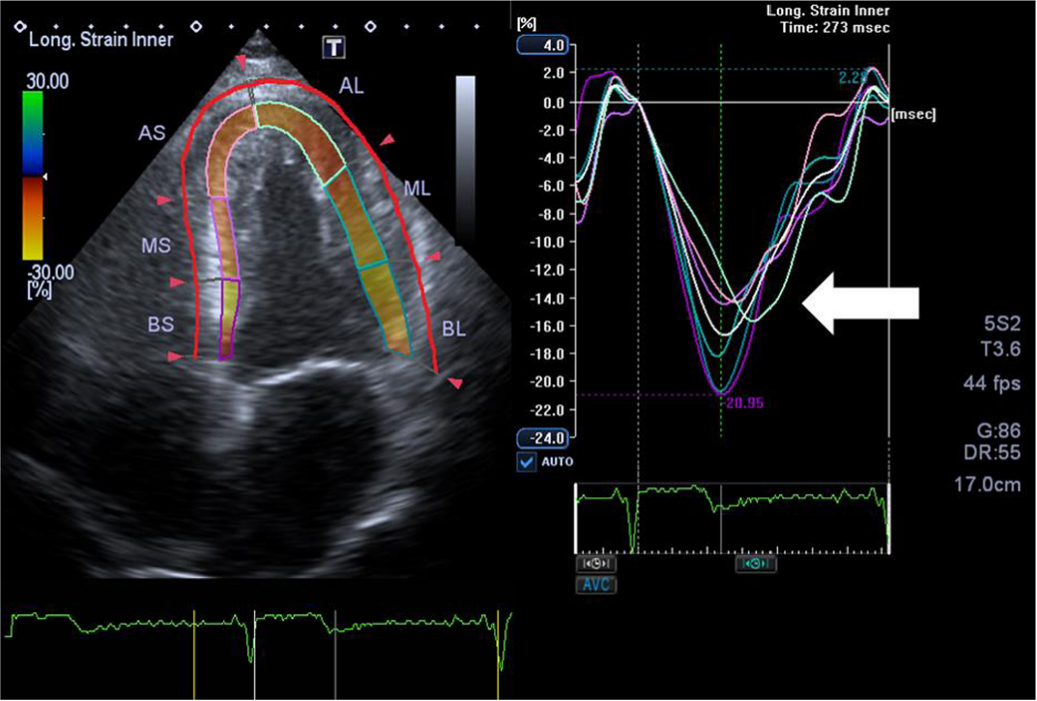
Endocardial longitudinal strain study of a patient with significant coronary artery disease. The automatic strain analysis in a patient with angina and significant left anterior descending (LAD) artery stenosis. The apical four-chamber view showes reduced colour coded subendocardial strain values segments supplied by the left LAD artery. Colour-coding from yellow to green indicates strain from +30% to -30%. Yellow = normal strain. Brown = areas with reduced strain. On the right strain curves for the 6 subendocardial segments are presented. The white arrow shows reduced strain values of – 14% in the curves representing the segments supplied by the LAD artery. AL = apicolateral; AS = apicoseptal; BL = basolateral; BS = basoseptal; ML = midlateral; MS = midseptal.
